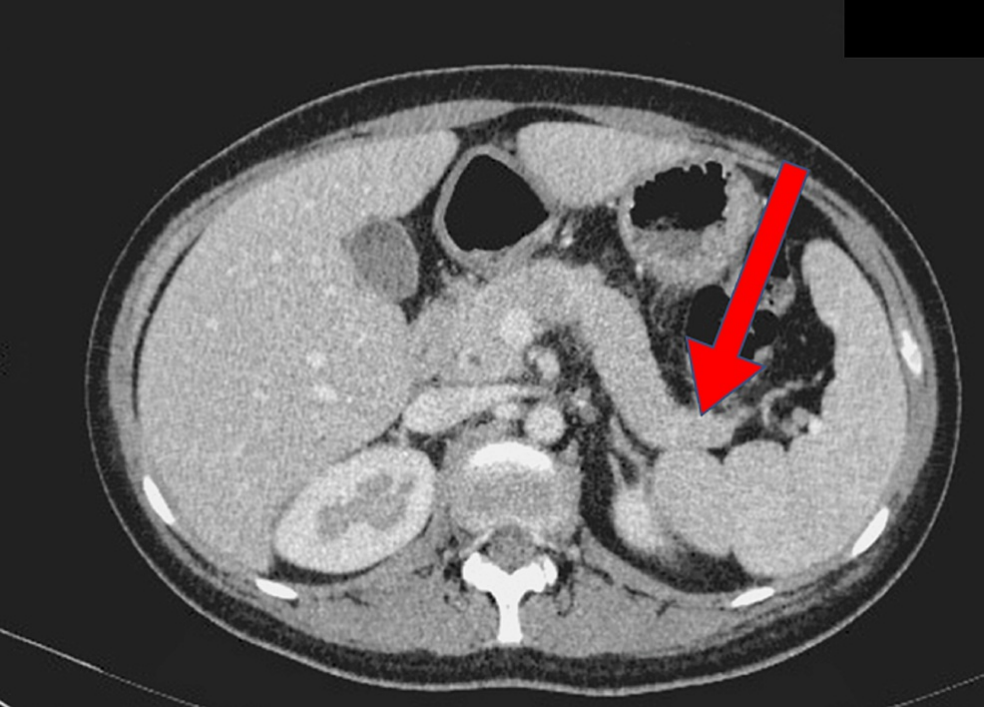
A repeated CT abdomen which showed resolution of pancreatitis (red arrow) CT: computer tomography
